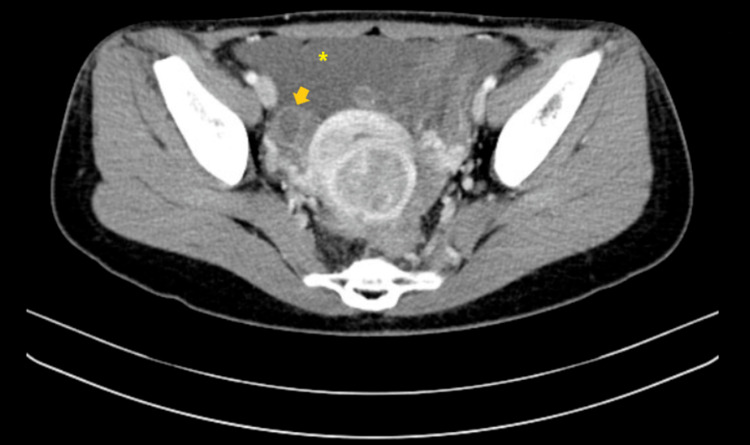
Abdominal CT scan – axial view showing massive ascites (asterisk), right adnexal mass (arrow), and soft tissue seeding CT: computed tomography
